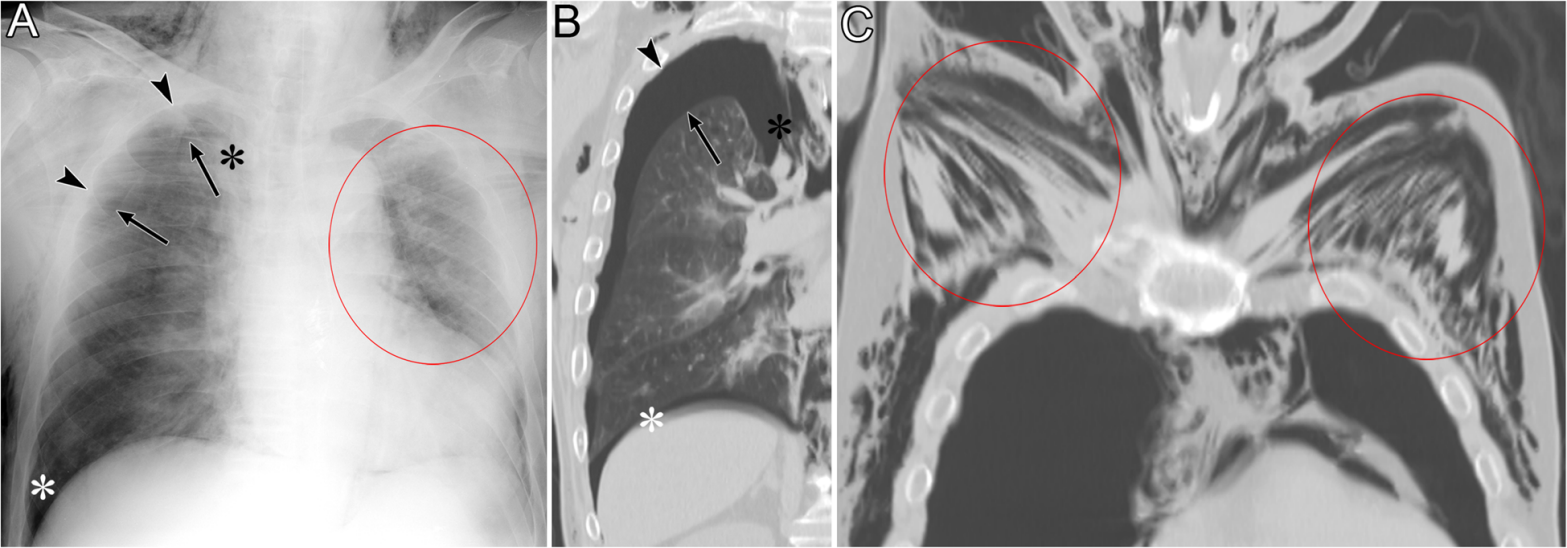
Pneumothorax in the apicolateral space of a 77-year-old man. Esophageal perforation caused by endoscopic submucosal dissection for esophageal cancer resulted in pneumomediastinum, pneumothorax, and subcutaneous emphysema. a A supine radiograph shows the visceral pleural line (black arrows) in the apicolateral space. The black arrowheads indicate the parietal pleura. Pneumothorax in the anteromedial space (black asterisk) and subpulmonary space (white asterisk), pneumomediastinum, and subcutaneous emphysema (red oval) are also visible. No vascular markings exist beyond the visceral pleural line. Subcutaneous emphysema in the pectoralis major muscle shows a linear disposition that follows the direction of the muscle fibers. b A coronal CT image reveals the visceral pleural line (black arrow) and the lack of any structures in the pleural cavity. The parietal pleura (arrowhead), pneumothorax in the anteromedial space (black asterisk), and pneumothorax in the subpulmonary space (white asterisk) are also observed. c A coronal CT image shows a linear disposition of subcutaneous emphysema that follows the direction of the muscle fibers of the pectoralis major muscle (red ovals)
